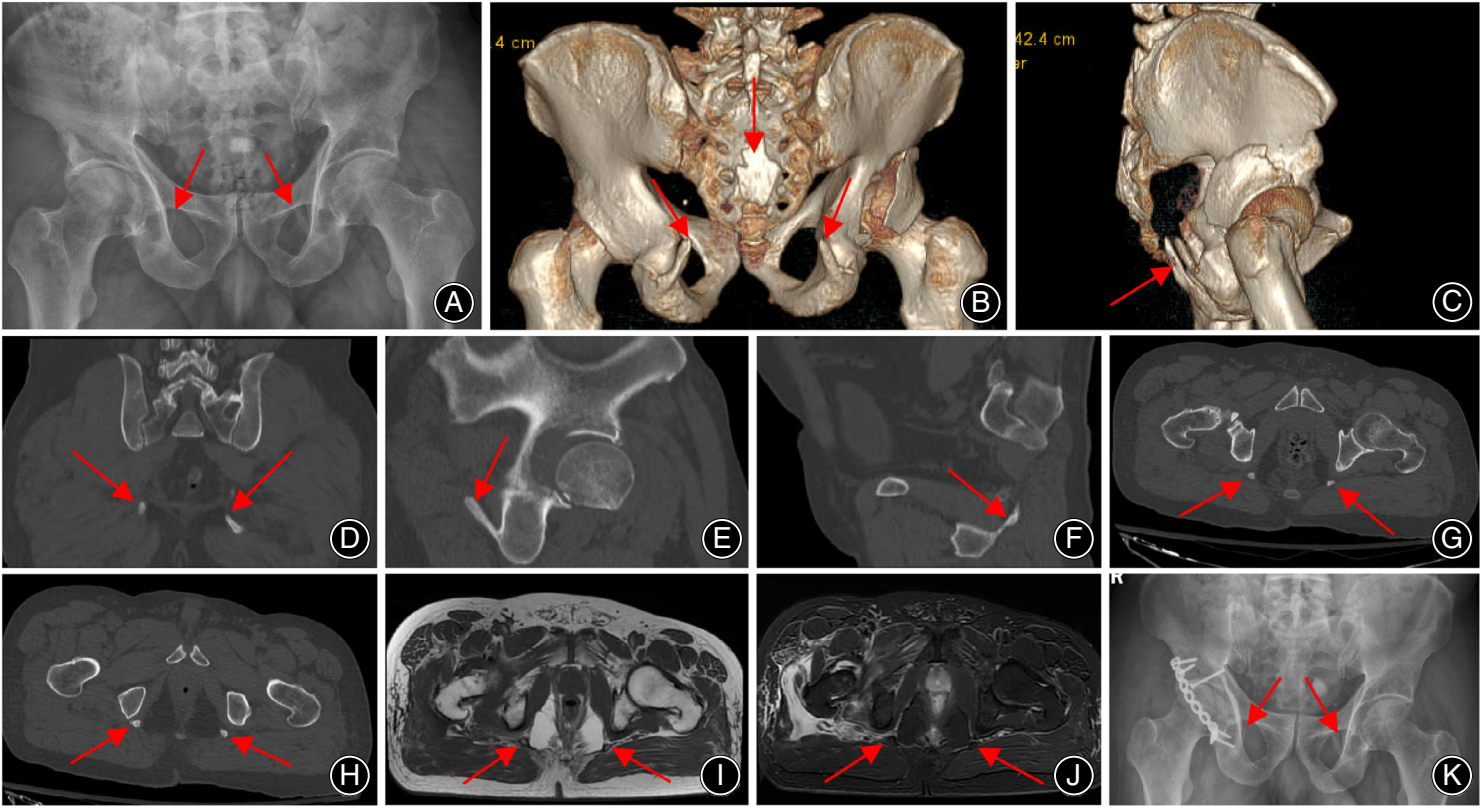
The patient's imaging data. (A) Anteroposterior view of pelvis on X‐ray displayed the injury of right hip joint and ossification of bilateral sacrotuberous ligaments. (B–H) CT scanning revealed typical signs of ossified sacrotuberous ligaments, with ossified signal observed at the attachment of the sacrum in three‐dimensional reconstruction. (I, J) Cross‐sectional views of T1 and T2 sequences of MRI showed the bilateral sacrotuberous ligaments. (K) Postoperative x‐ray image.
